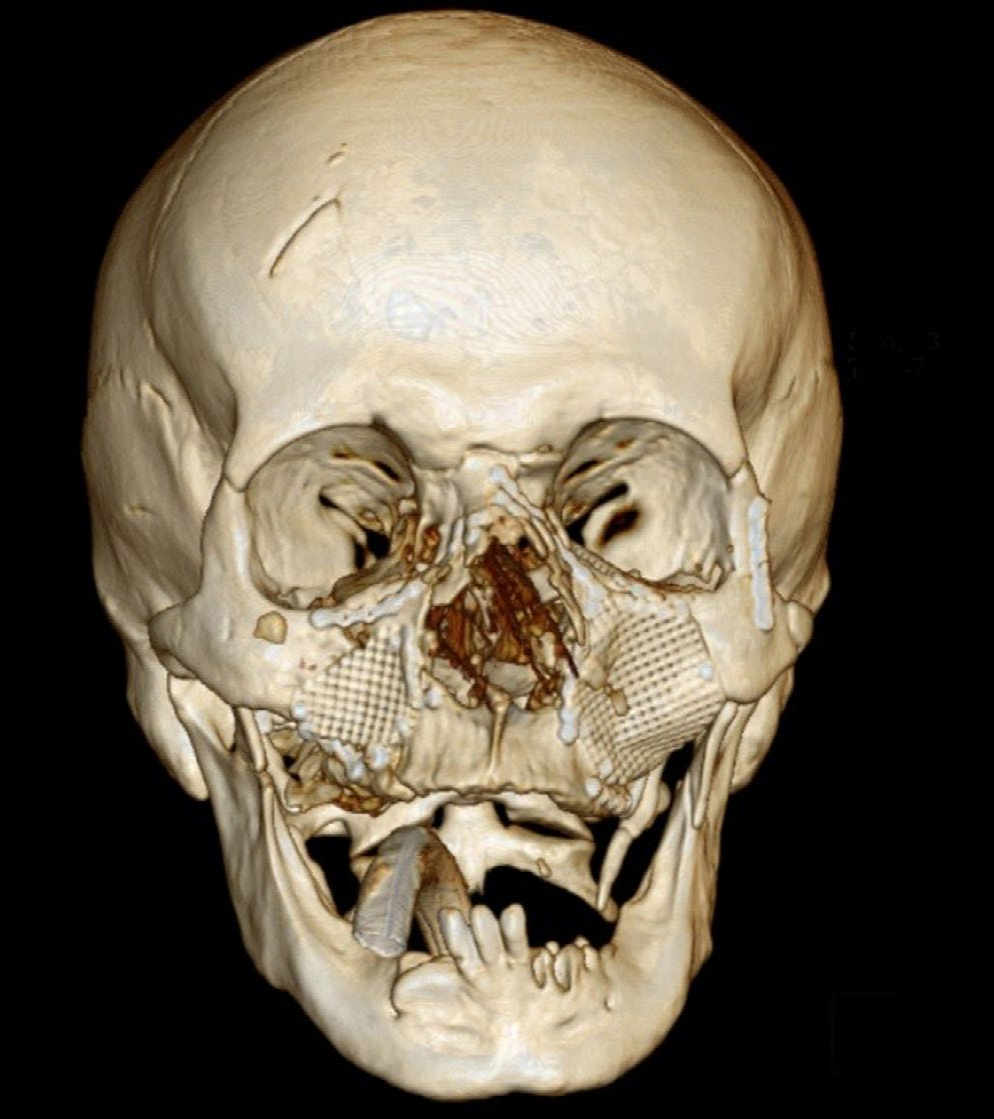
Immediate three‐dimensional CT scan depicting reduction of fractures using miniplates and screws and utilization of titanium mesh for reconstruction of orbital floor and anterior walls of maxillary sinuses
